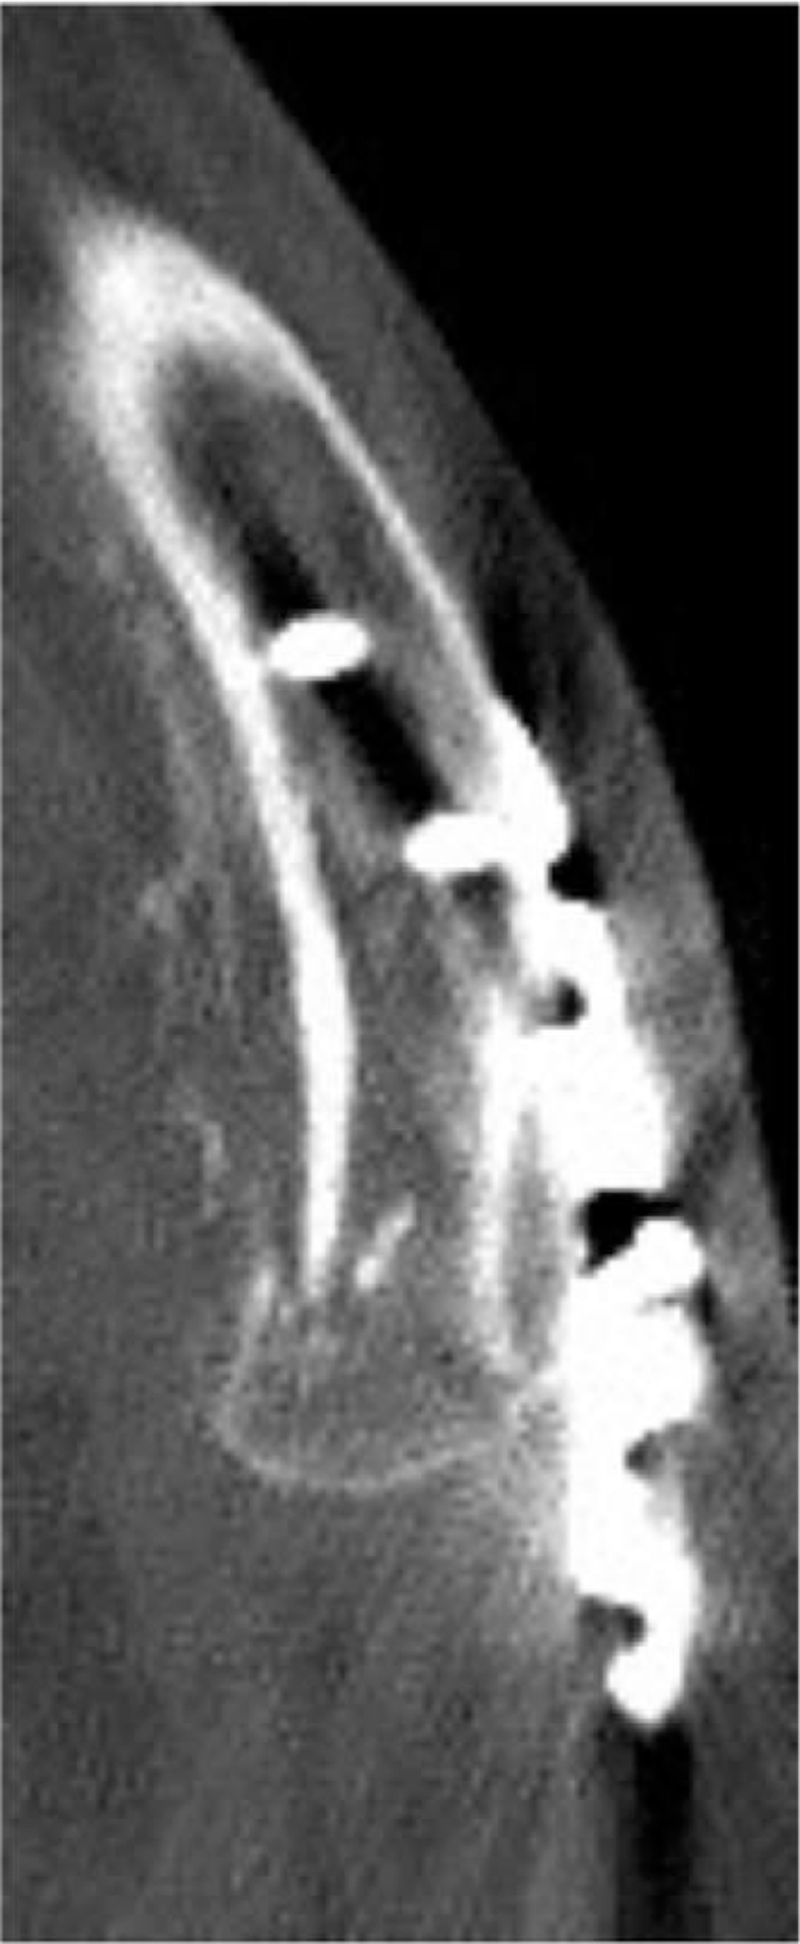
CT scan showed satisfactory healing of lateral clavicle fractures with fuzzy fracture line and the callus formation (CT = computed tomography).
